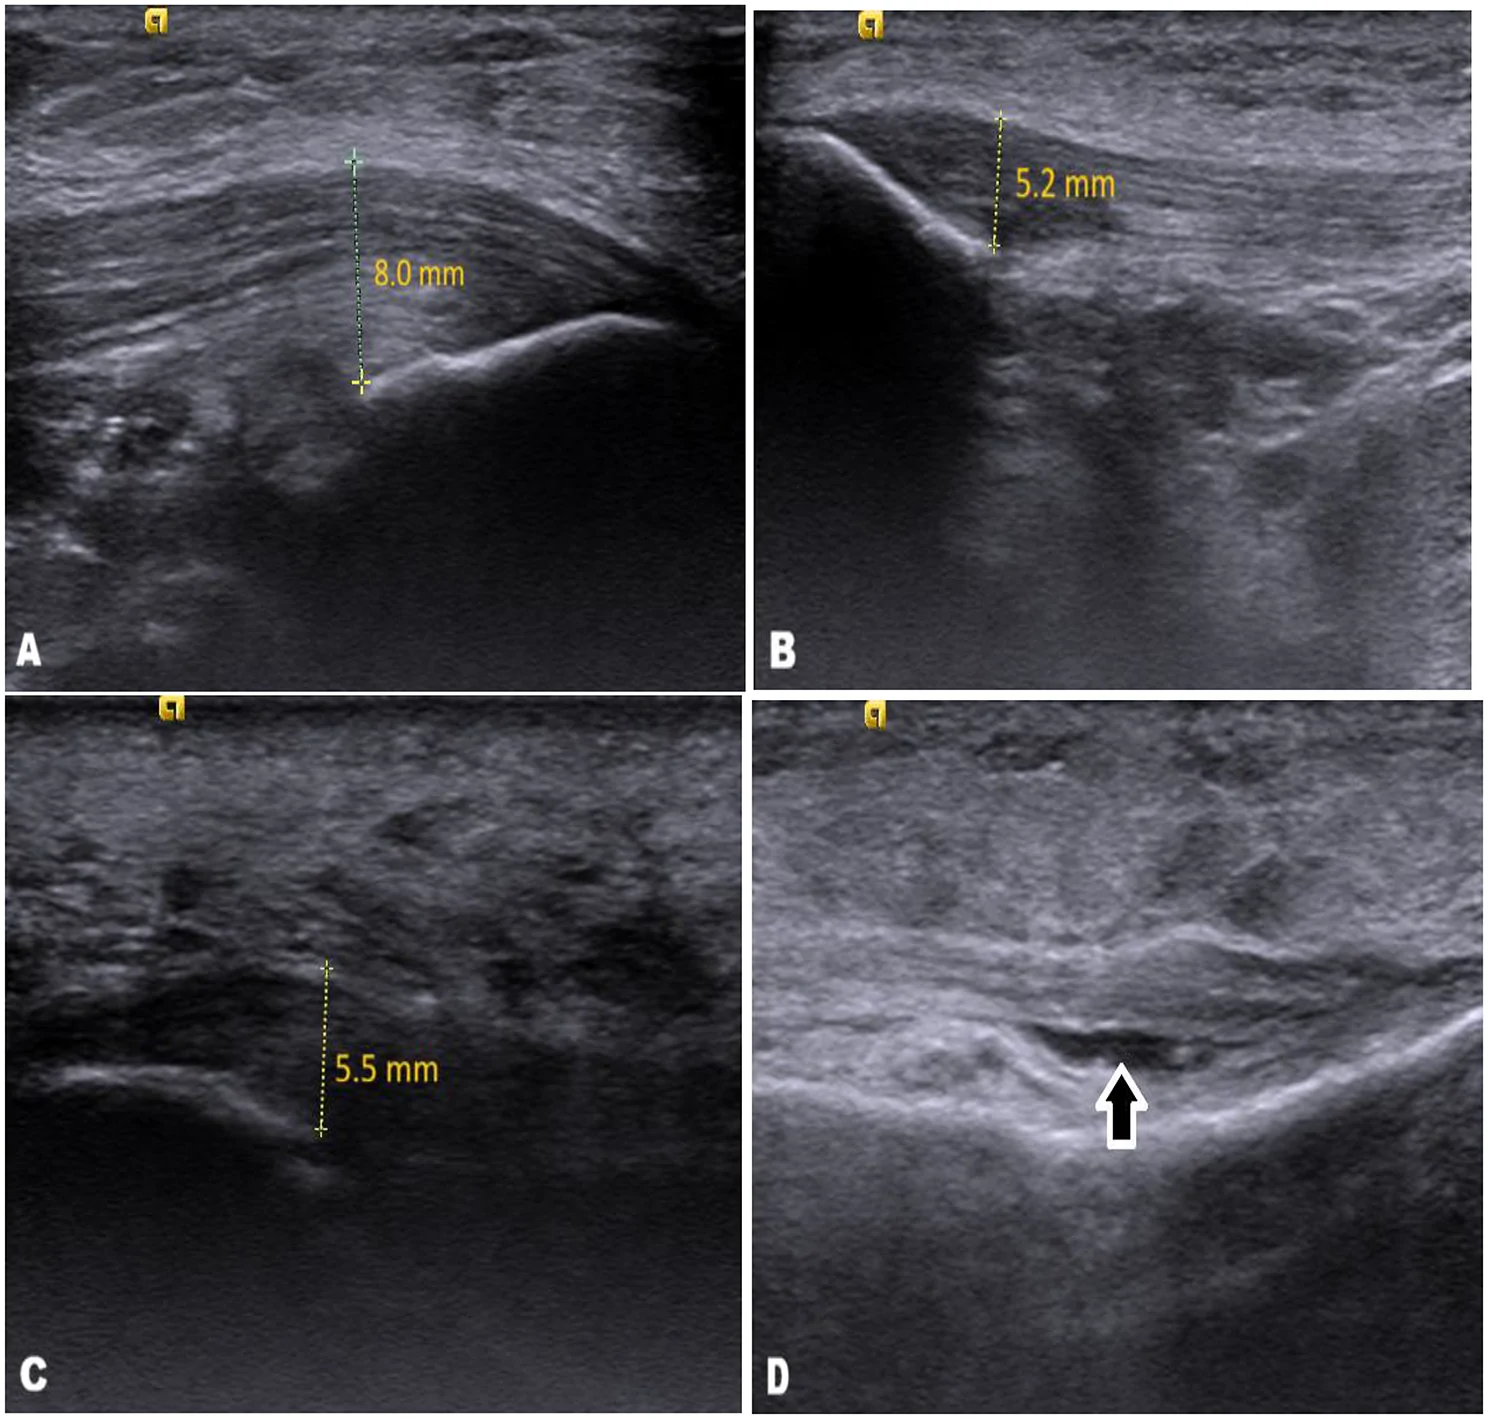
Representative gray-scale ultrasound images demonstrating structural entheseal abnormalities in patients with type 2 diabetes mellitus. (A) Quadriceps tendon showing significant thickening (8.0 mm) at the superior patellar insertion. (B) Proximal patellar tendon thickening (5.2 mm) at the inferior patellar pole. (C) Plantar fascia thickening (5.5 mm) at the calcaneal origin. (D) Longitudinal scan revealing anechoic fluid collection (arrow) consistent with pes anserine bursitis. Note: Thickness measurements were obtained strictly at the entheseal insertion sites in accordance with the GUESS protocol
